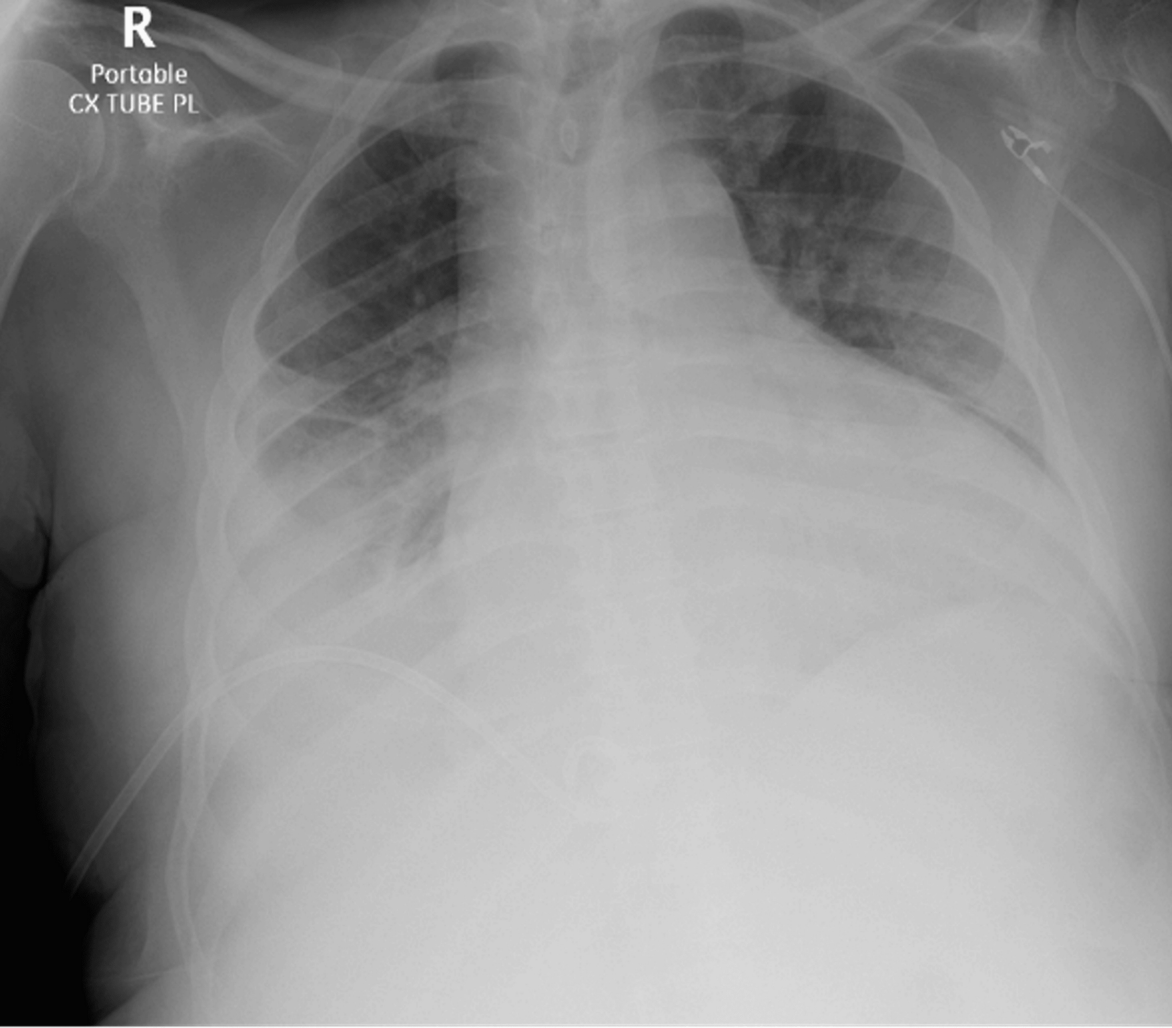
Chest X-ray demonstrates a moderate right pleural effusion.
